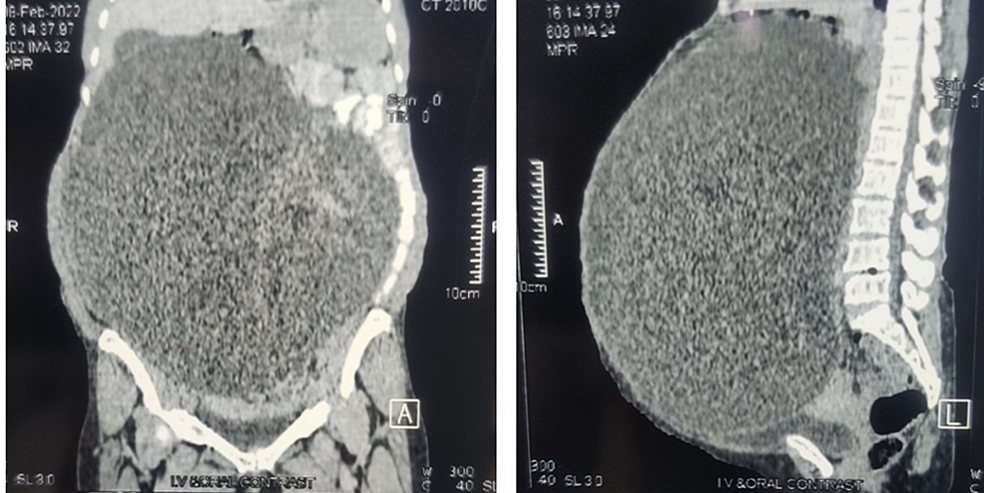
Multidetector CT of abdomen, sagittal (left) and axial (right) view showing mutiloculated cystic lesion arising from right ovary with thick septation *CT- Computed Tomography
